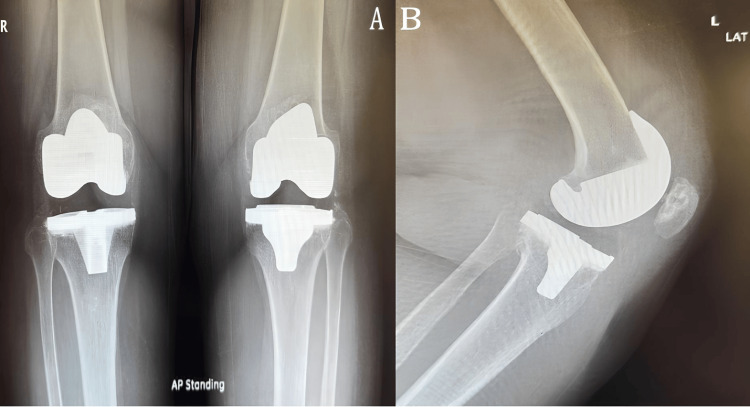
(A) Bilateral standing knee AP view X-ray two months postoperatively of the left side and 11 months operatively of the right side. (B) Left lateral view AP: anteroposterior
